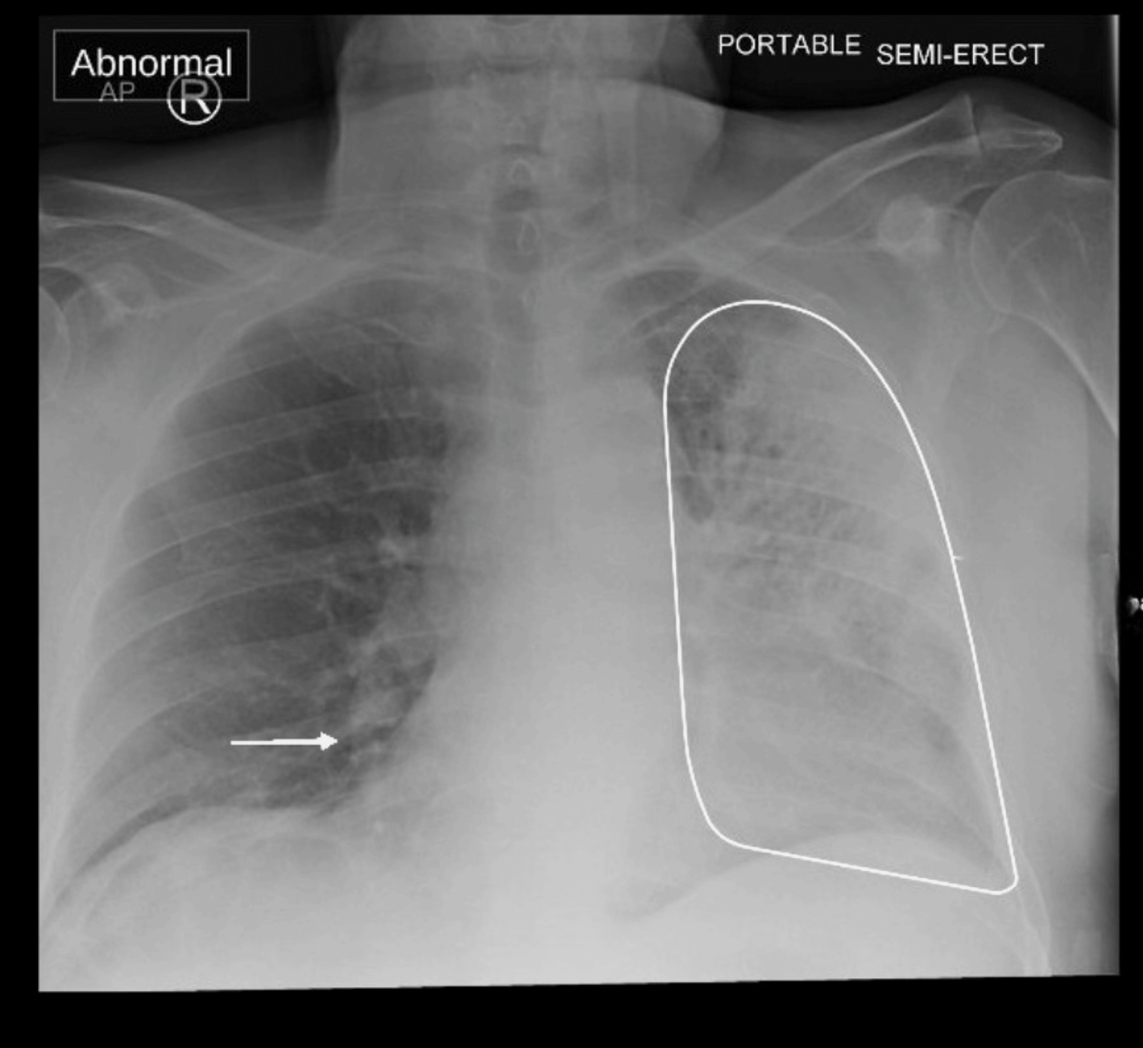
Chest radiograph on admission
